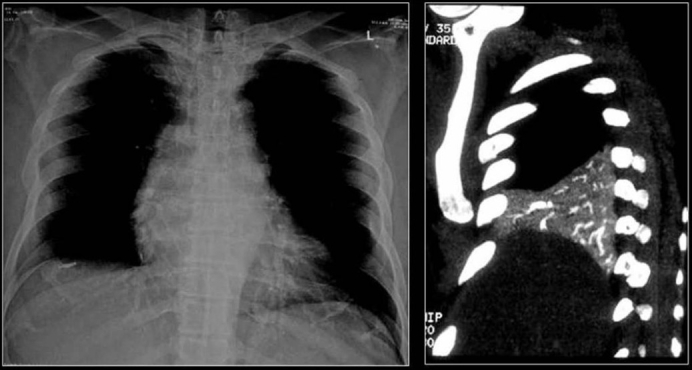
A chest radiograph and coronal reconstruction of CT shows tubular opacities of metallic density due to acrylic cement pulmonary emboli as a complication of vertebroplasty
